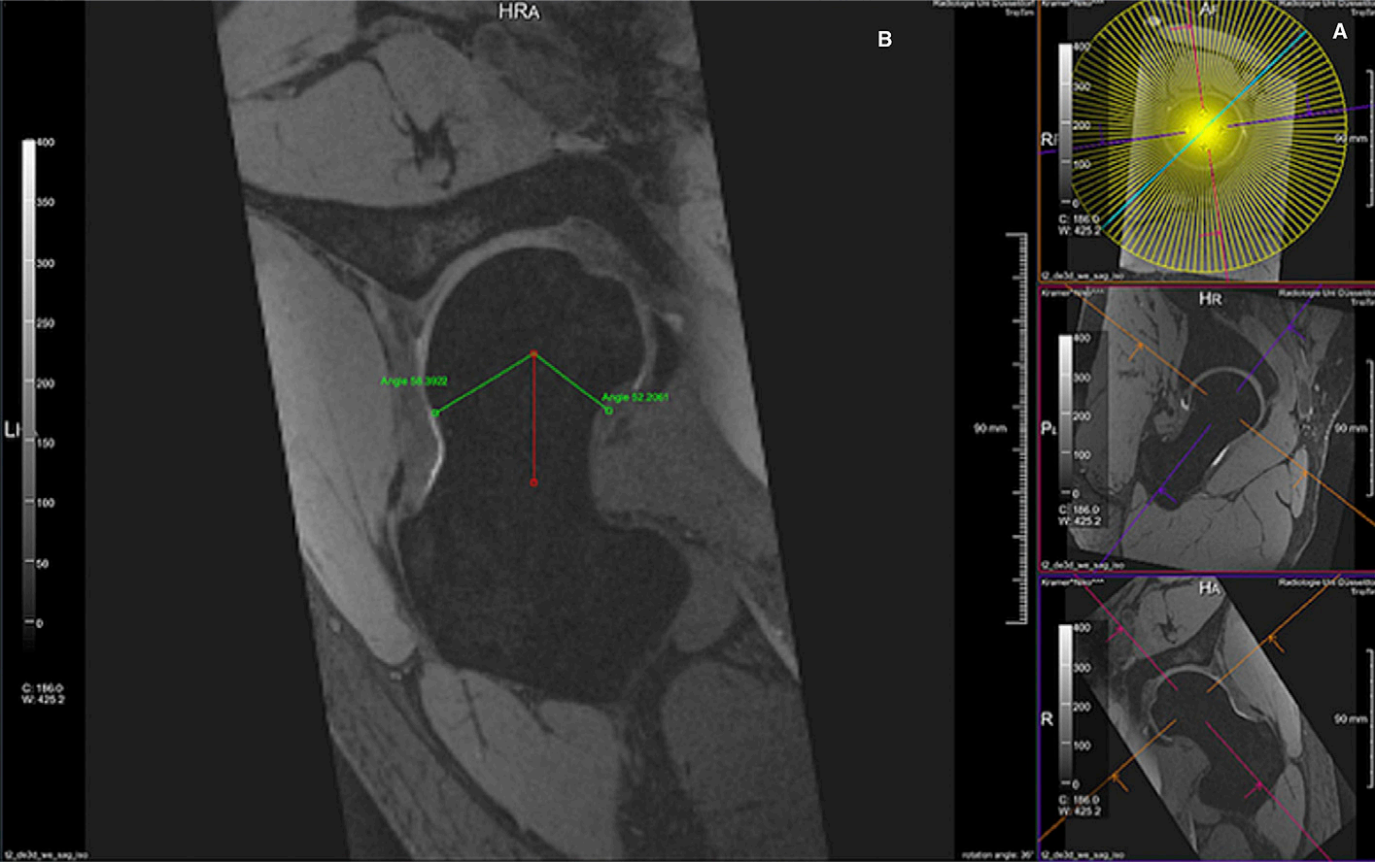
Alpha angle measurement using automated computer-based software (MR Chondral Health 2.1; Siemens Healthcare). Fig. 4-A There were 120 radial planes that could be used for alpha angle measurement. Fig. 4-B Alpha angle measurement performed by the software. (Reproduced, with labeling added, from: Ewertowski NP, Schleich C, Abrar DB, Hosalkar HS, Bittersohl B. Automated measurement of alpha angle on 3D-magnetic resonance imaging in femoroacetabular impingement hips: a pilot study. J Orthop Surg Res. 2022 Jul 30;17[1]:370, under a Creative Commons Attribution 4.0 International License.)
